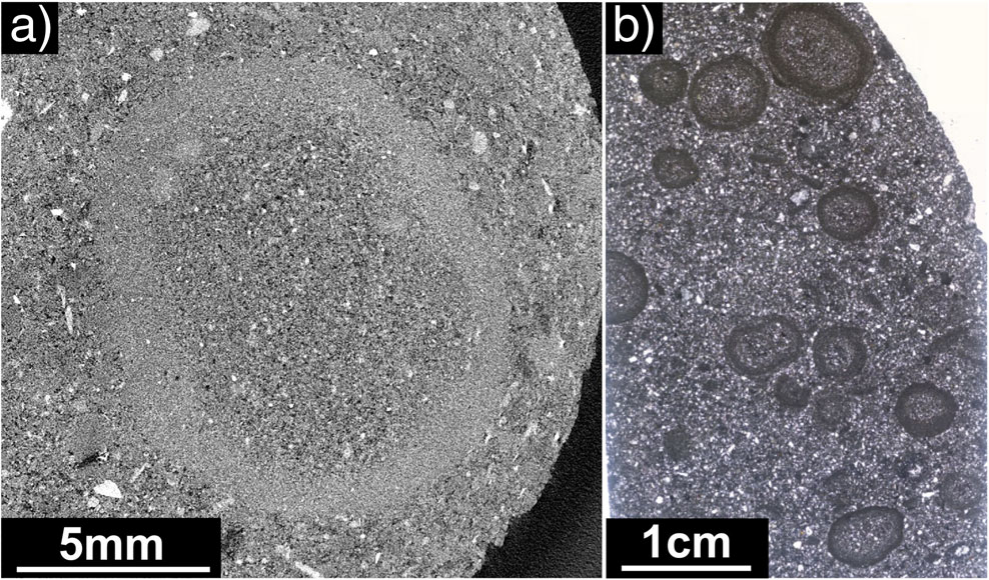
a X-ray CT image of an AP2 type sample from the FBN73 drill core inside the Nördlinger Ries, Germany, sampled at a depth of 296 m (see also Stoeffler et al. 2013). The aggregate is about 1.1 cm in diameter and has an overall PSD < 150 μm with a clearly finer PSD of about <85 μm in the rim. b Accumulation of AP2 type aggregates, also from the FBN73 drill core but sampled at a depth of 313 m. Aggregates are between 0.5 and 1 cm in size and show clear coarse-grained core and fine-grained rim features
